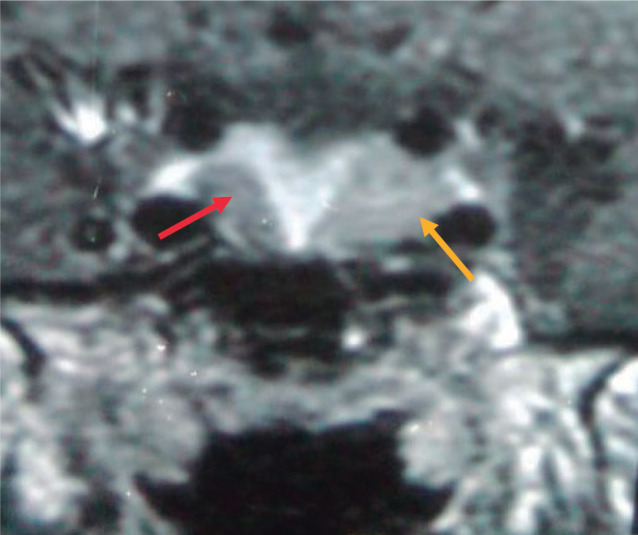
Magnetic resonance imaging demonstrating two distinct pituitary adenomas.
